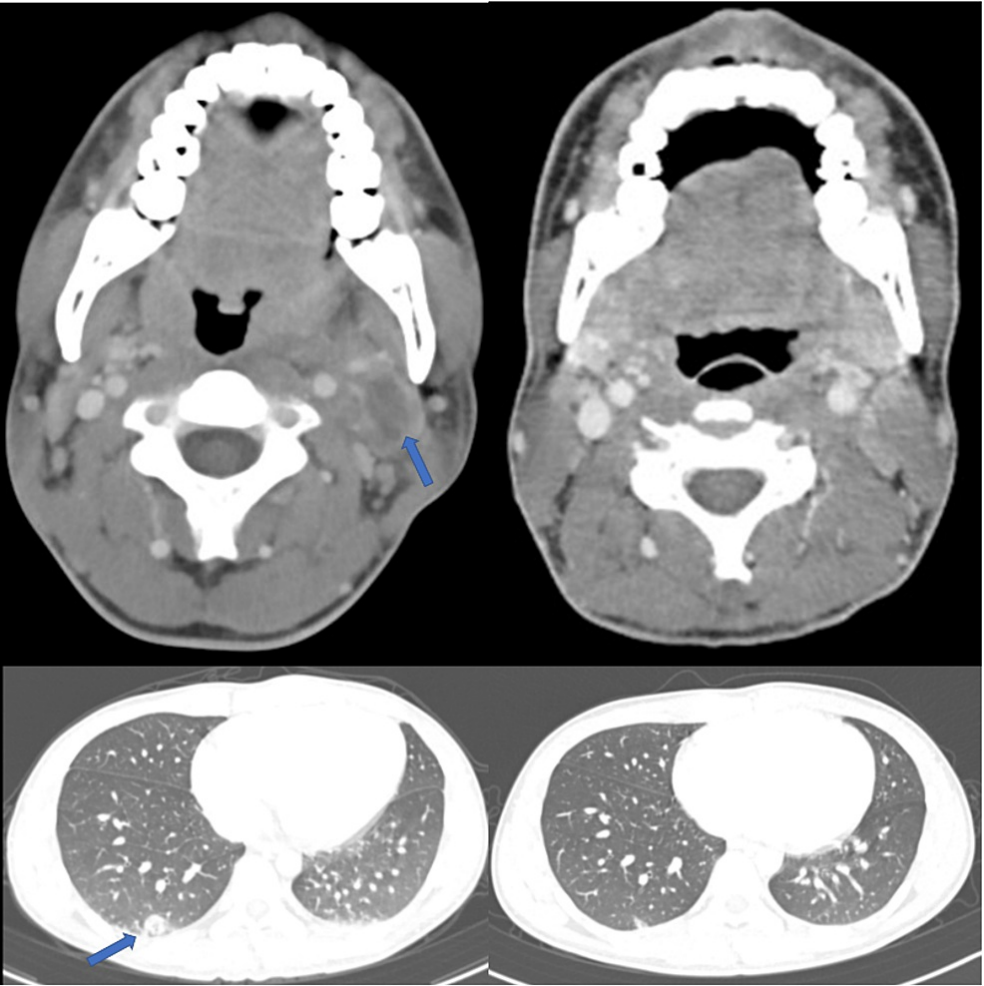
Serial computed tomography (CT) findings Left: Contrast-enhanced computed tomography (CT) of the neck performed on day 1. The upper left image shows a thrombus in the left internal jugular vein. The contrast-enhancing effect suggests abscess formation in the soft tissue around the internal jugular vein (arrows). The image on the lower left shows a round nodule suspected to be a septic embolus (arrows). Right: Contrast-enhanced CT of the cervical region on day 59. The image on the upper right shows the resolution of the neck abscess. The image on the lower right shows the resolution of the pulmonary nodule.
